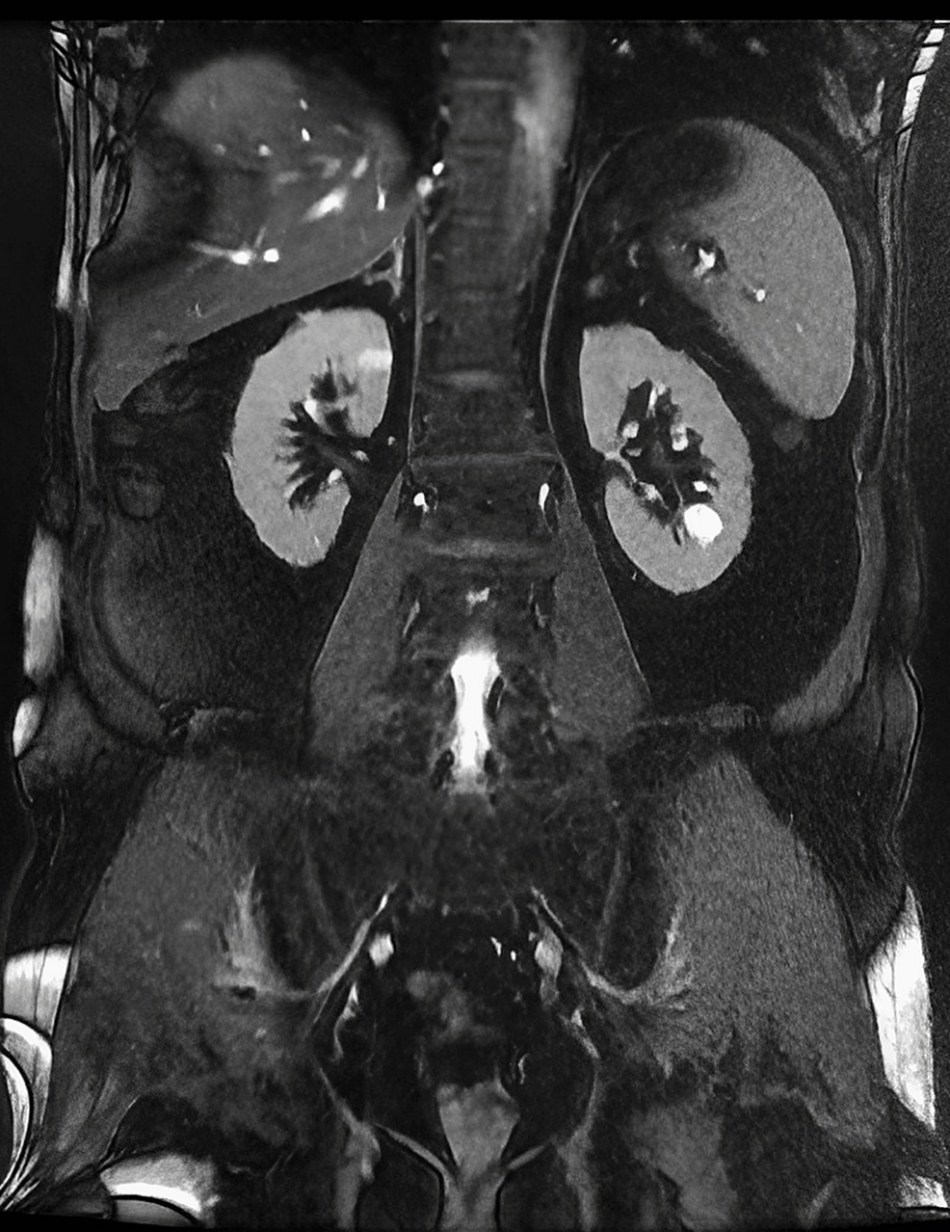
MRI - abdomen Screening of the abdomen using MRI T2-weighted images demonstrates no other discrete lesions noted elsewhere MRI: magnetic resonance imaging
